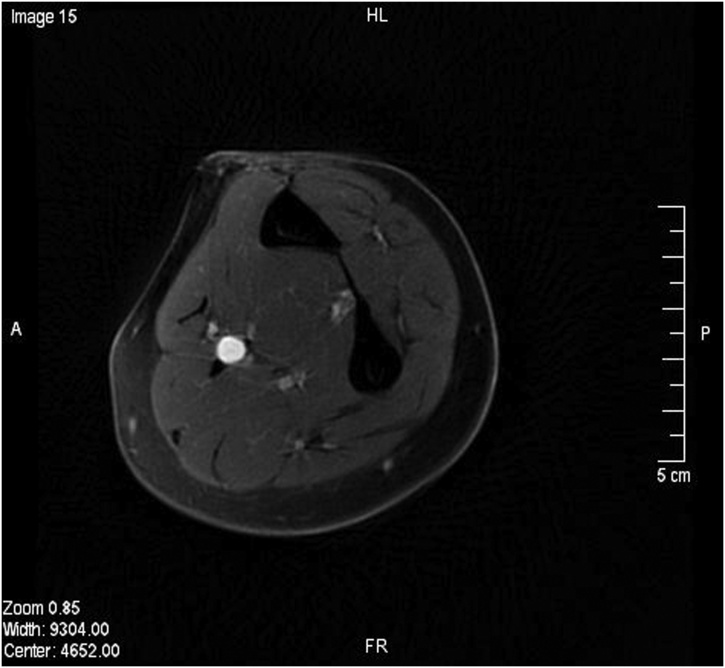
Axial MRI of the forearm showing ulnar artery aneurysm.
